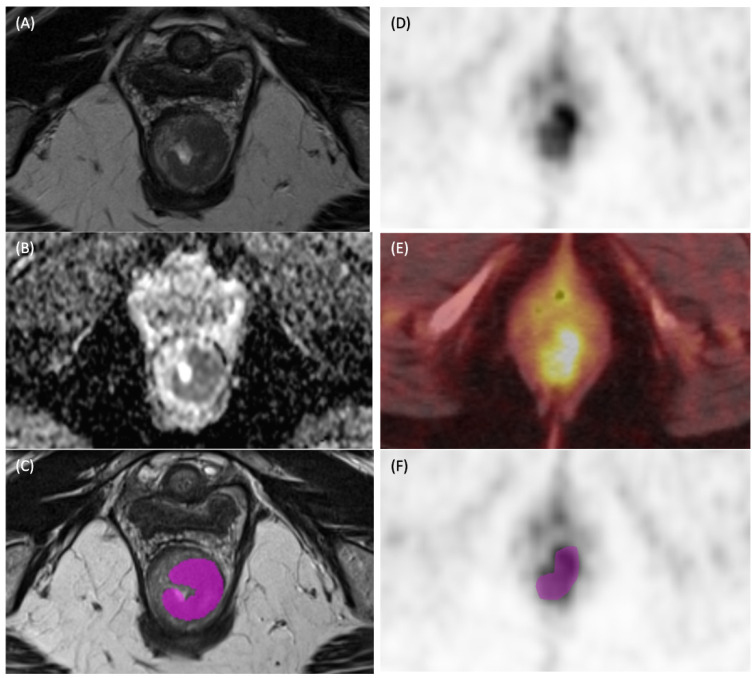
Representative images of tumor segmentation in a patient with anal squamous cell carcinoma. (A) Axial T2-weighted MRI image (T2WI) demonstrating the primary intermediate T2 signal intensity tumor with almost circumferential involvement of the anal canal. (B) Corresponding ADC map. (C) Axial T2WI showing corresponding manually contoured region of interest (ROI). (D) 18F-FDG PET Maximal intensity projection (MIP) image showing corresponding increased metabolic activity in the tumor. (E) Fused PET/CT image. (F) Corresponding manually contoured region of interest.
